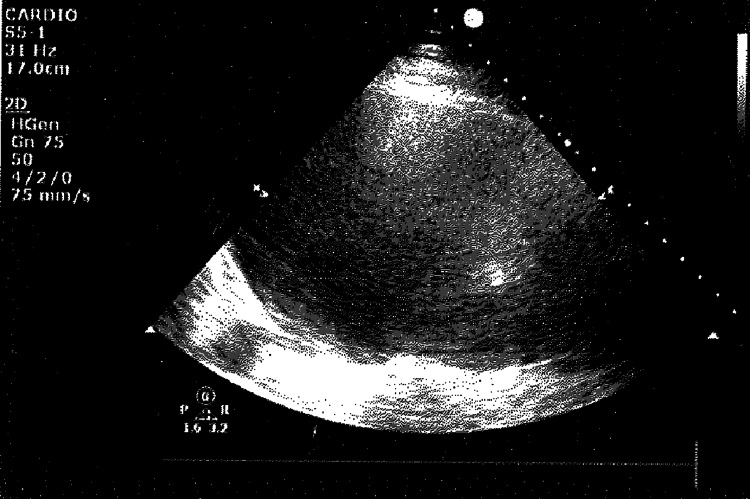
The left-sided black pleural effusion as seen on ultrasound prior to drainage
